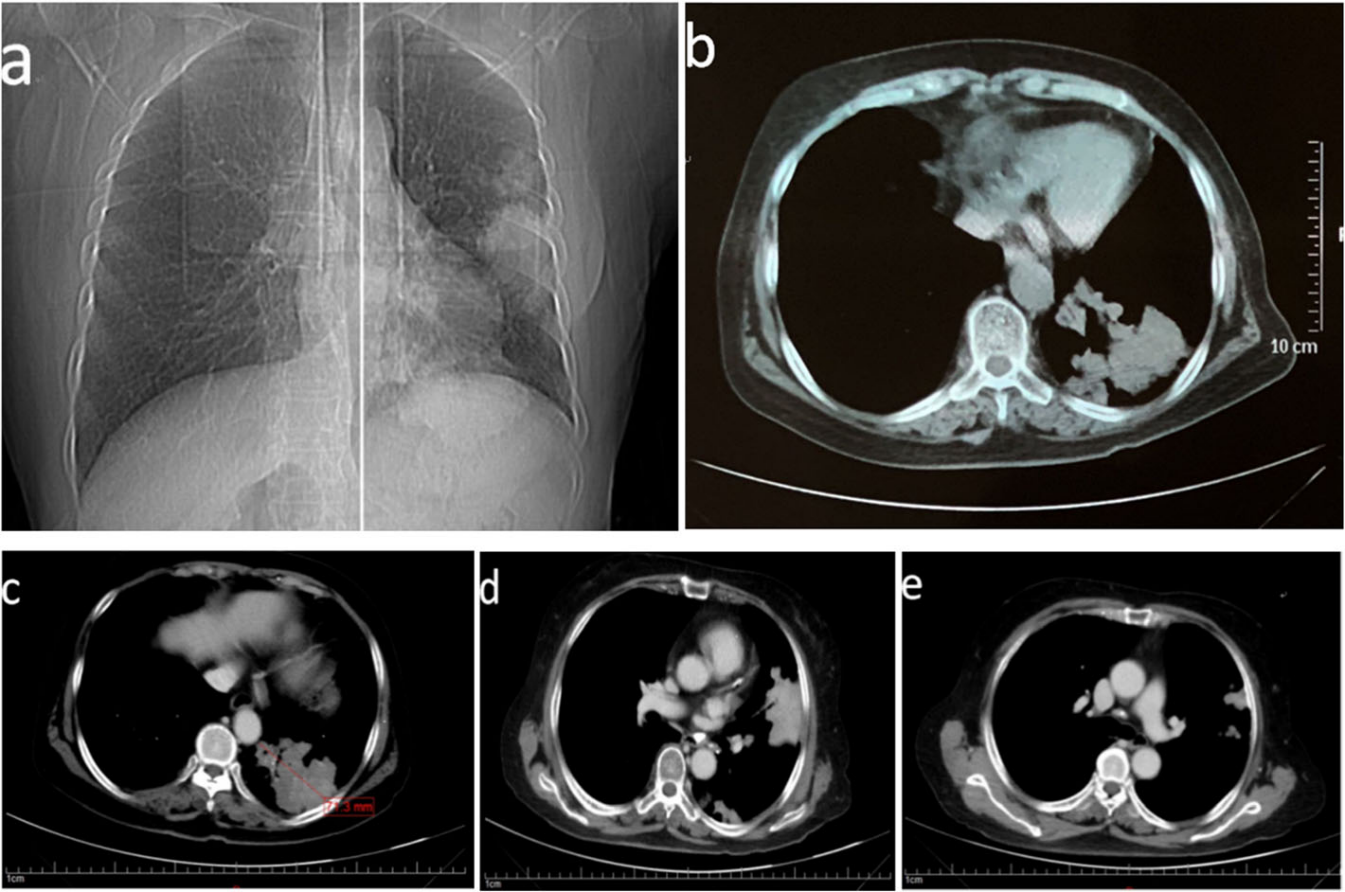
Imaging examinations: a Chest radiography:nodules in the upper and lower segments of the left lung. b CT: “cauliflower-like tumor” formed by fusion of multiple nodules. c CT: soft tissue mass with diameter of 71.3 mm. d CT: multinodular confluent tumor close to the pleura. e Some small nodules in the lungs
